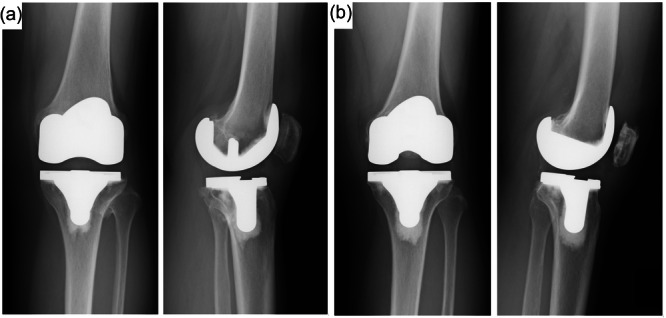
Anteroposterior and lateral plain radiographs of two types of TKA 1 year post‐operatively. (a) MP‐TKA and (b) PS‐TKA have the same shape except for the presence of the box. MP, medial‐pivot; PS, posterior‐stabilized; TKA, total knee arthroplasty.
